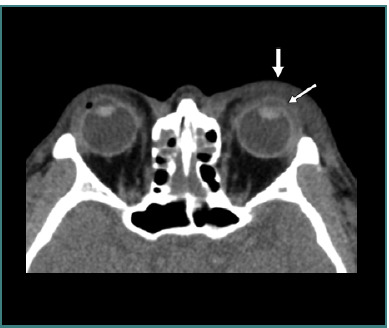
Axial computed tomography scan without contrast of left globe (false positive open globe). The thin arrow represents wall deformity (clinical examination showed subconjunctival hemorrhage and edema) and the thick arrow represents eyelid hematoma. Image reproduced with permission from the American Roentgen Ray Society: Yuan WH, Hsu HC, Lin YY, Liao SW. CT of globe rupture: analysis and frequency of findings. AJR Am J Roentgenol. 2014;202(5):4-5. ^©^ 2014 American Roentgen Ray Society.
